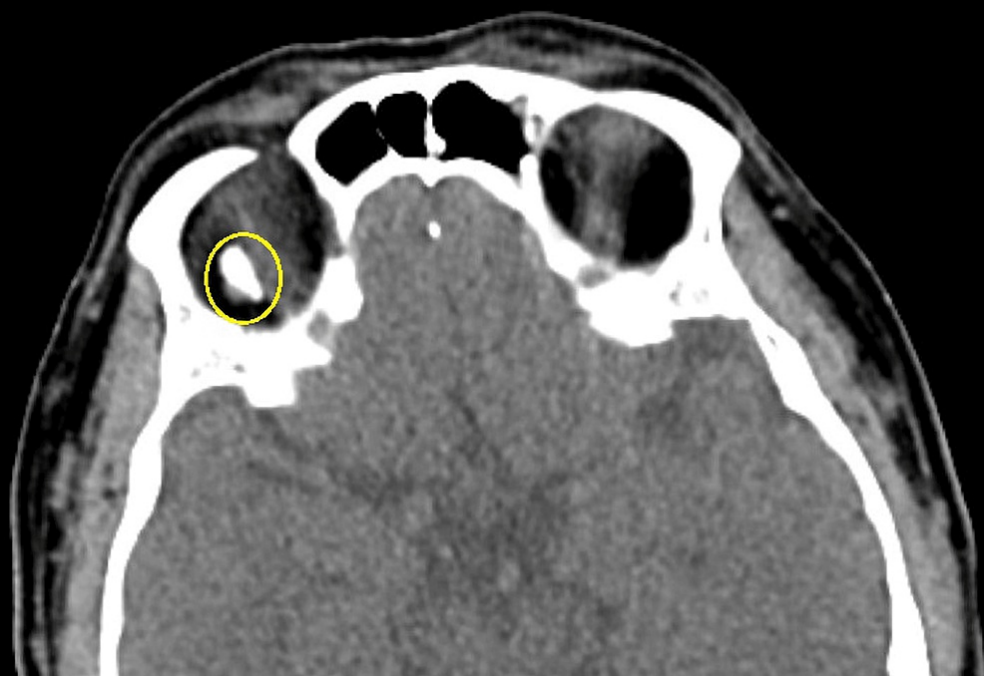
Axial view of contrast-enhanced computed tomography orbit scan showing a hyperdense foreign material situated in intraconal space (yellow circled) without surrounding tissue reaction and not encapsulated.
